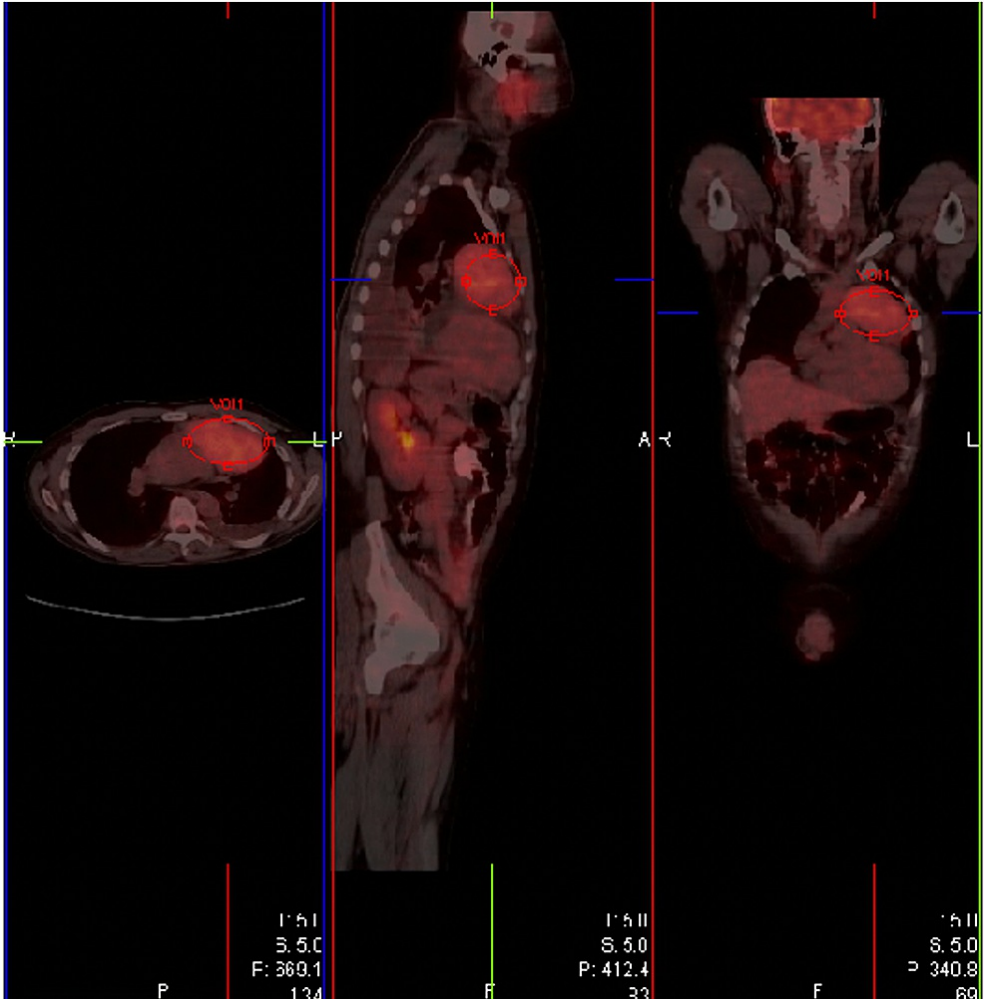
PET-CT showing heterogenous increased uptake localized to an approximately 9.5 x 7 cm soft tissue mass in the anterior mediastinum. SUV max 4.3. SUV: standardized uptake value
